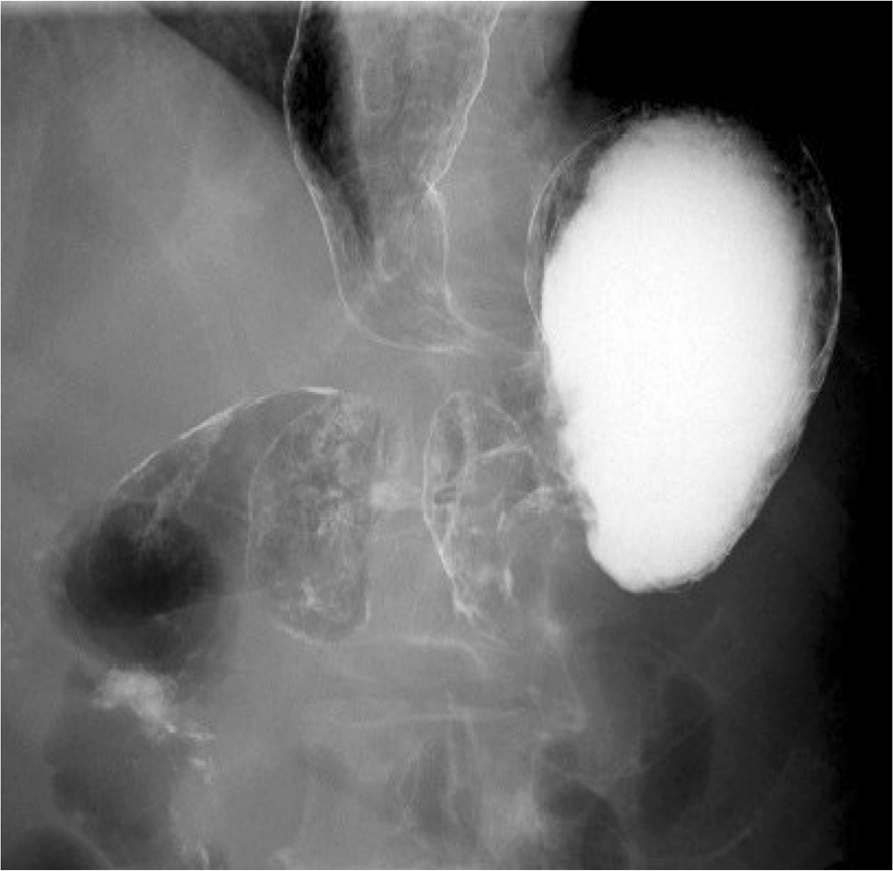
Contrast radiography after the fourth cycle of first-line chemotherapy. Contrast radiography revealed two sections of stenosis in the esophago-gastric junction and gastric body. The length of the stenosis from the gastric lower body to the antrum was 4cm, and the pyloric ring was intact.
